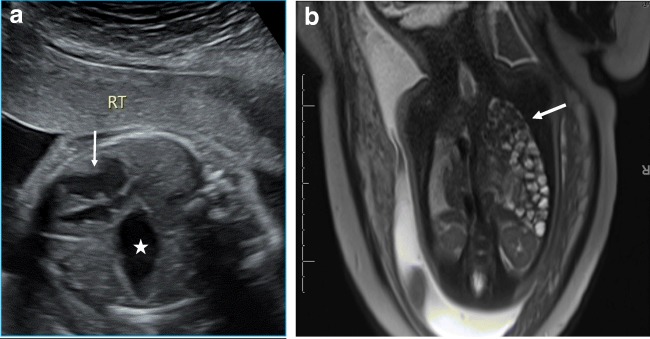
a Axial ultrasound image of a 21-week-old foetus identifies stomach (star) at the level of the heart (white arrow) in the thorax. This finding is suggestive of a congenital diaphragmatic hernia. b Coronal images as seen on prenatal MRI (b) demonstrates multiple loops of fluid-filled bowel (white arrow) extending to the left thoracic apex, compatible with a Bochdalek hernia. There is associated ipsilateral lung hypoplasia
